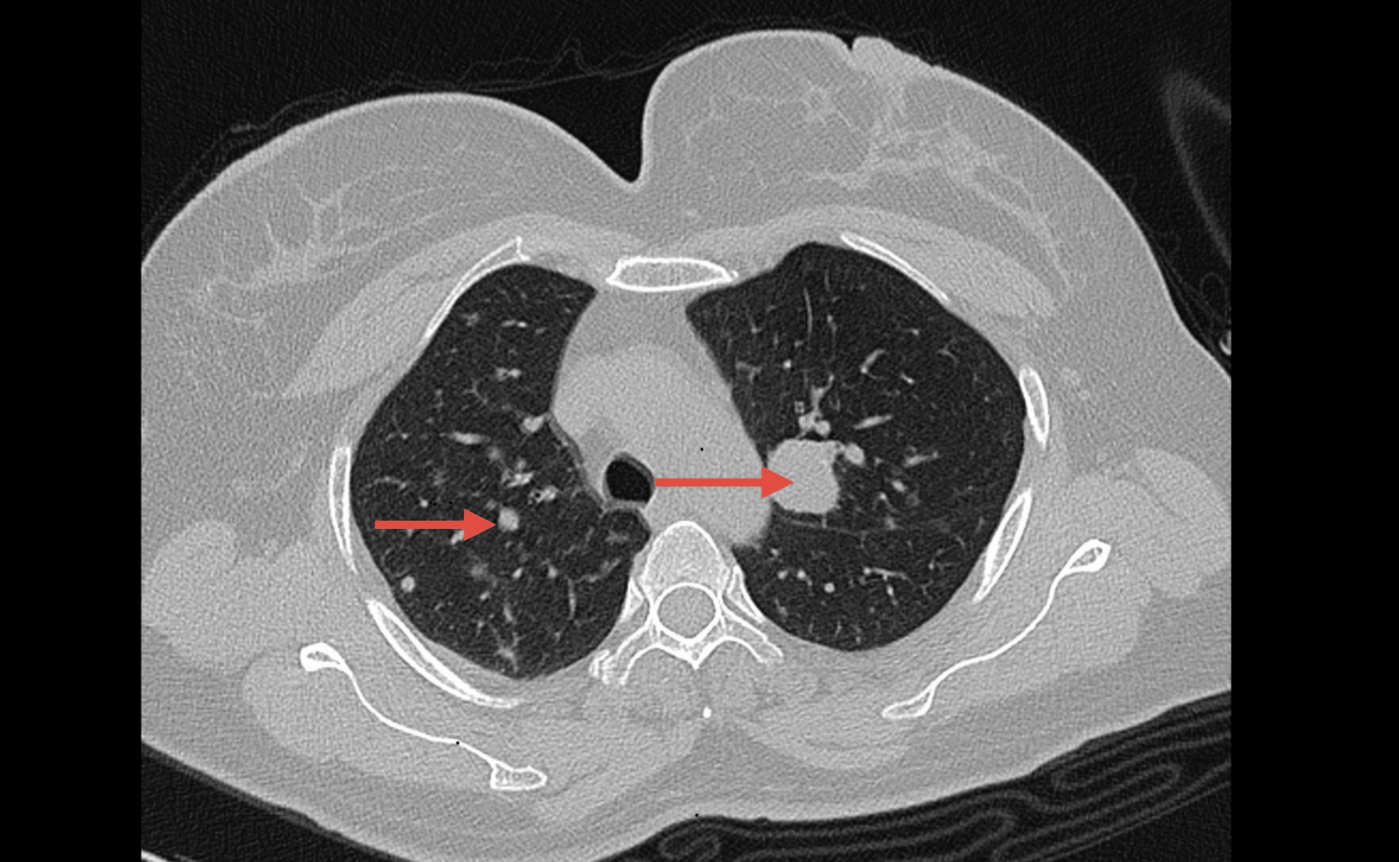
Cross-sectional image of a chest CT scan Arrow indicates multiple round opacities disseminated in both lung fields.
